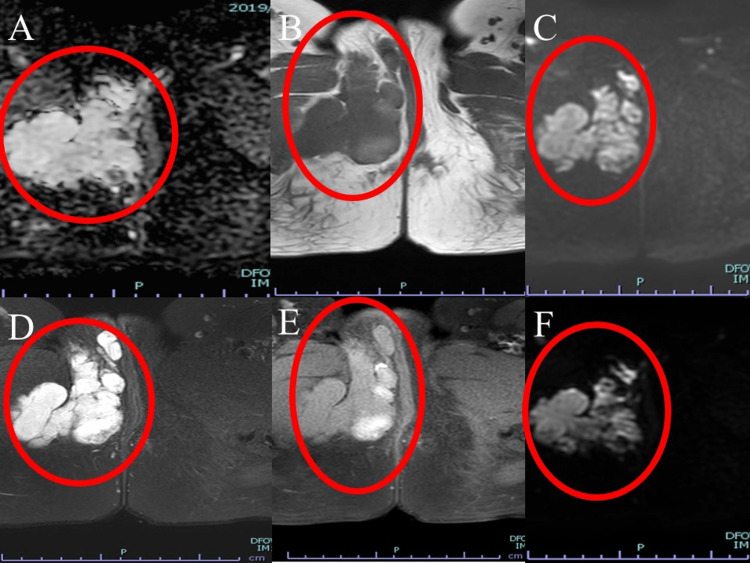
Magnetic resonance images. Various types of axial dissection. The T1-weighted image showed a mild hypointense signal. Diffusion-weighted images (DWI) showed a hyperintense signal, and apparent diffusion coefficient (ADC) maps showed a hypointense signal. A is an ADC image. B is a T1-weighted image. C is a diffusion-weighted image with a b-value of 1000s/mm^2^. D is a fat-suppressed T2-weighted image. E is a fat-suppressed T1-weighted image. F is a diffusion-weighted image with a b-value of 0s/mm^2^.
